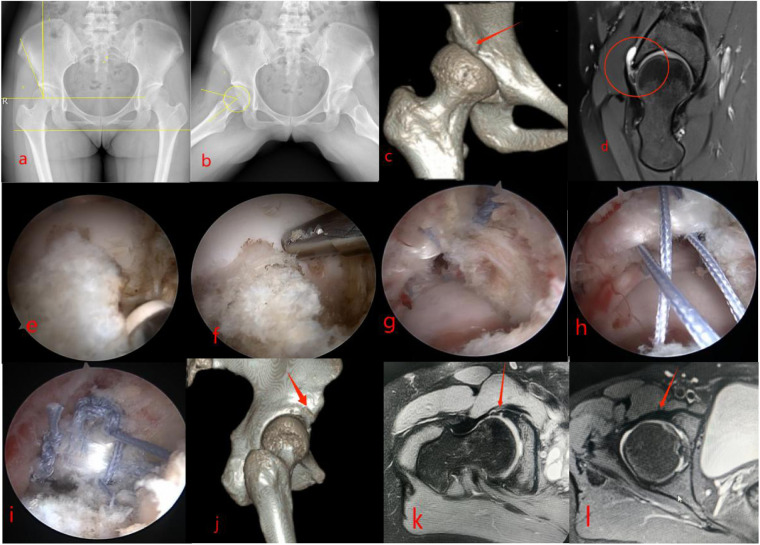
Representative case of arthroscopic labral repair, femoroacetabular osteoplasty, and capsular reconstruction in a 28-year-old female with symptomatic acetabular labral tear and borderline hip dysplasia (BDDH). Preoperative assessment (a–d): (a) Anteroposterior pelvic radiograph shows lateral center-edge angle (LCEA) 20.5°, consistent with BDDH. (b) Dunn view radiograph reveals alpha angle 55°, indicating mild cam morphology. (c) CT scan demonstrates type II anterior inferior iliac spine (AIIS) impingement. (d) MRI shows a paralabral cyst with anterior labral base avulsion. Intraoperative arthroscopic procedure (e–i): (e) T-shaped capsulotomy using radiofrequency ablation device. (f) Arthroscopic shaver debrides redundant inferior extracapsular tissue to prepare for capsular plication. (g) Medial capsular closure with interrupted sutures. (h) Lateral capsular reconstruction using V-shaped plication technique. (i) Arthroscopic view confirming watertight capsular closure. Postoperative follow-up (j–l): (j) CT at 24 h shows satisfactory AIIS contouring. (k) Immediate MRI demonstrates anatomical capsular coaptation. (l) 6-month MRI confirms complete capsular healing without redundancy.
